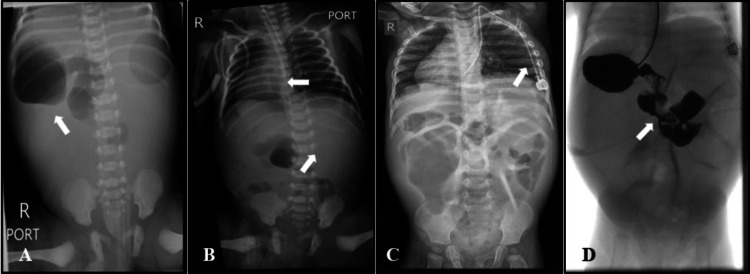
Radiographic imaging 1A: plain abdominal X-ray revealing right-sided gastric shadow; 1B: plain chest and abdominal X-ray showing right-sided cardiac shadow and left-sided hepatic shadow (situs inversus totalis); 1C: postoperative plain chest and abdominal X-ray showing a port-a-cath central line in place and gaseous distension of the bowels; 1D: postoperative Gastrografin study to assess bowel continuity and the total length
